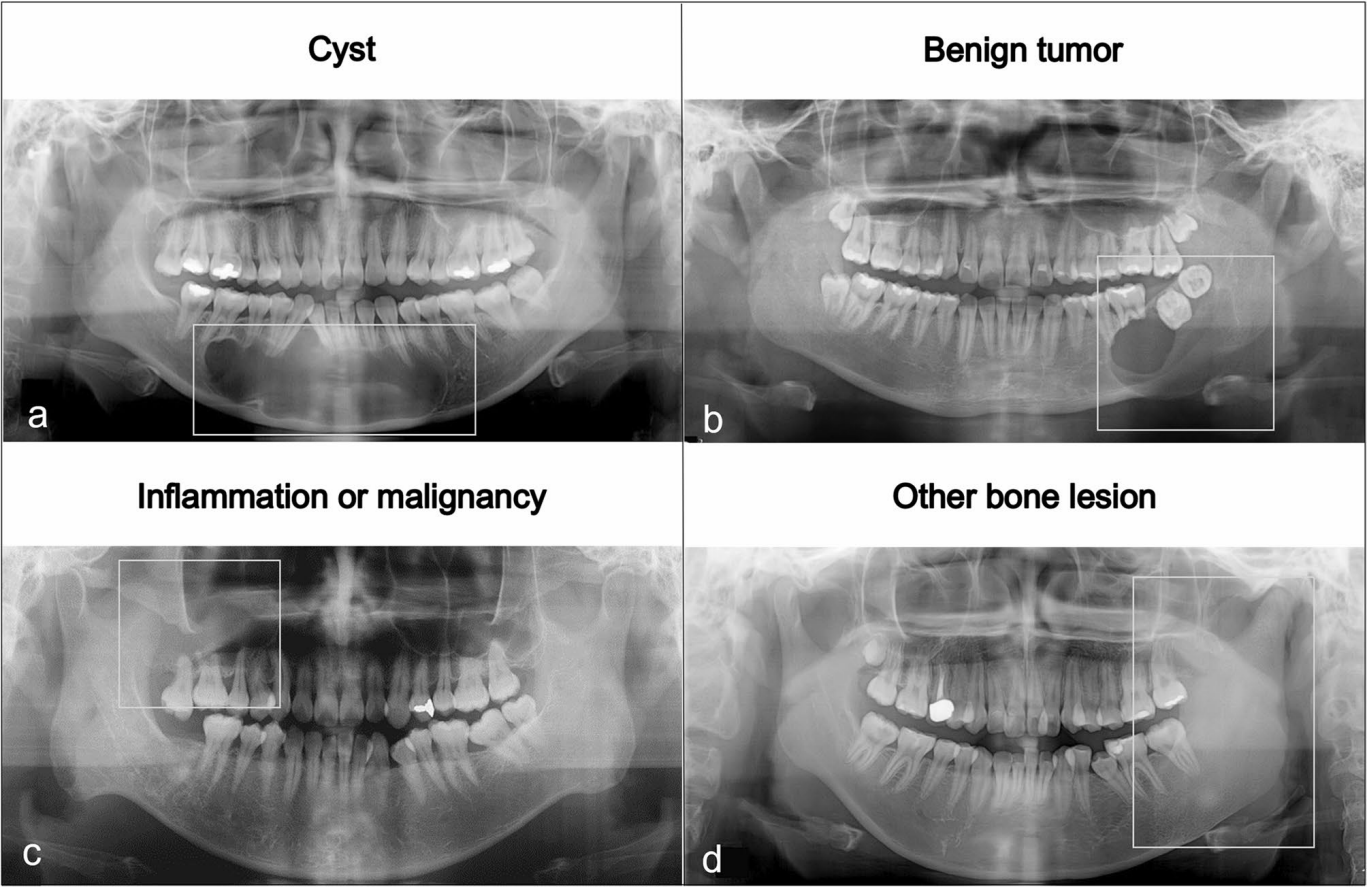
Examples of four categories of jaw lesions. Cyst (a), benign tumor (b), inflammation or malignancy (c), and other bone lesion (d). Jaw lesions are presented in white boxes on each panoramic radiograph
